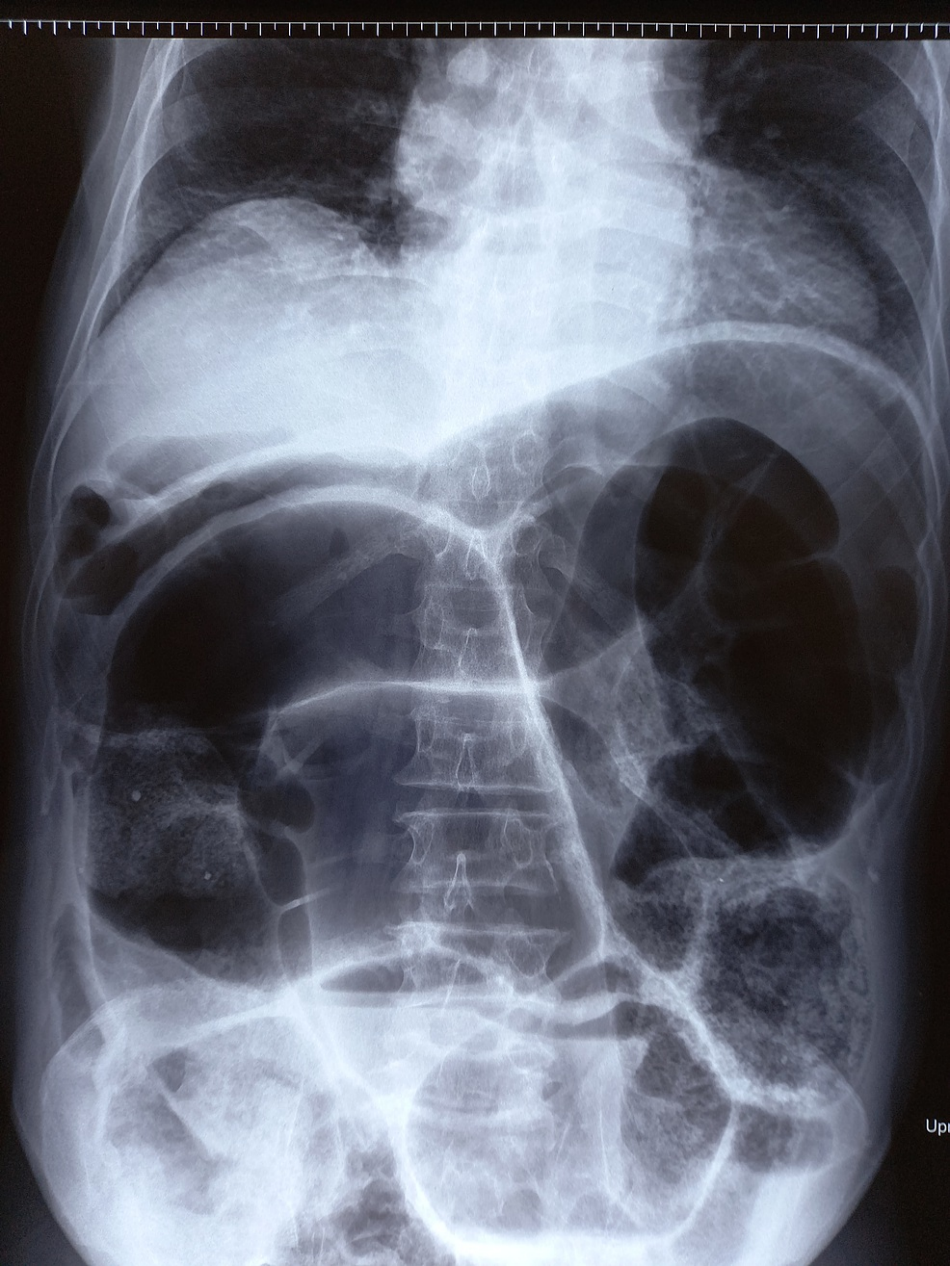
X-ray of the abdomen showing a distended large bowel.
